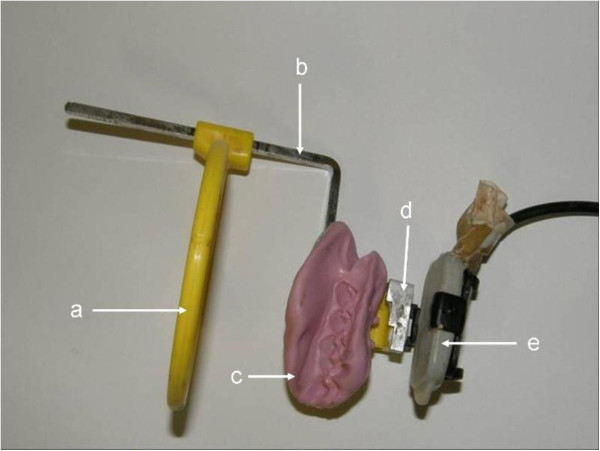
The apparatus after a lab putty impression was made. This impression was made to ensure that images were acquired in the same position every time an image of that quadrant was made. (a + b = Rinn apparatus; c = #21 mandible disposable impression tray with Lab Putty impression; d = aluminum step wedge; e = Gendex Visualix EHD Digital intra-Oral x-ray unit – Size 1 censor (universal size) attached to a XPC-DS Digital Position System.
